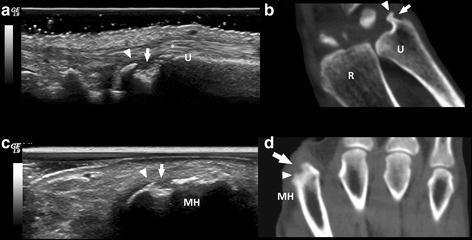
Bone erosions in patients with Jaccoud’s arthropathy. Longitudinal ultrasound (US) image of the lateral ulnar head area (*U*) showing a grade-2 scoring ultrasound structural erosion (ScUSSe) (*arrow*) with a hook-shaped deformity (*arrow head*) (a). *U* ulnae, *R* radial, *extensor carpi ulnaris tendon. Coronal computed tomography (CT) scan of the wrist showing a grade-4 rheumatoid arthritis magnetic resonance imaging score (RAMRIS) erosion (*arrow*) confirming the hook-shaped deformity (*arrow head*) with a bony sclerotic margin (b). Transversal US view of the second metacarpophalangeal (MCP) dorsal area showing a grade-3 ScUSSe hook-shaped (*arrow head*) erosion (*arrow*) (c) corresponding to a grade 3 RAMRIS score erosion (*arrow*) with hook-shaped deformity (*arrow head*) visible in the CT coronal scan of the hand (d). *MH* second metacarpal head
